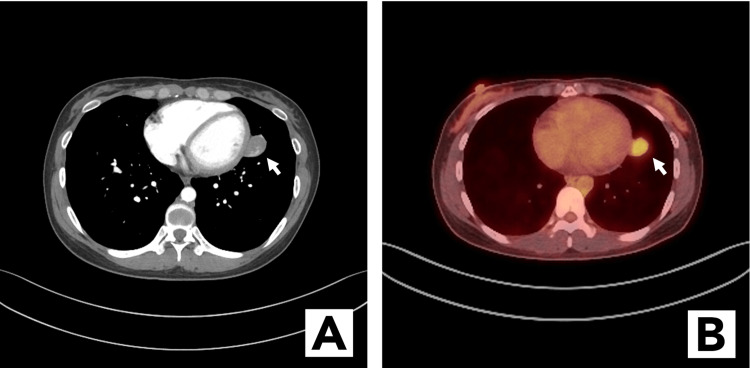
(A) CT showing a nodule in the lingula abutting the major fissure. (B) PET-CT image with FDG-avid showing uptake in the nodule. PET, positron emission tomography; FDG, fluorodeoxyglucose
